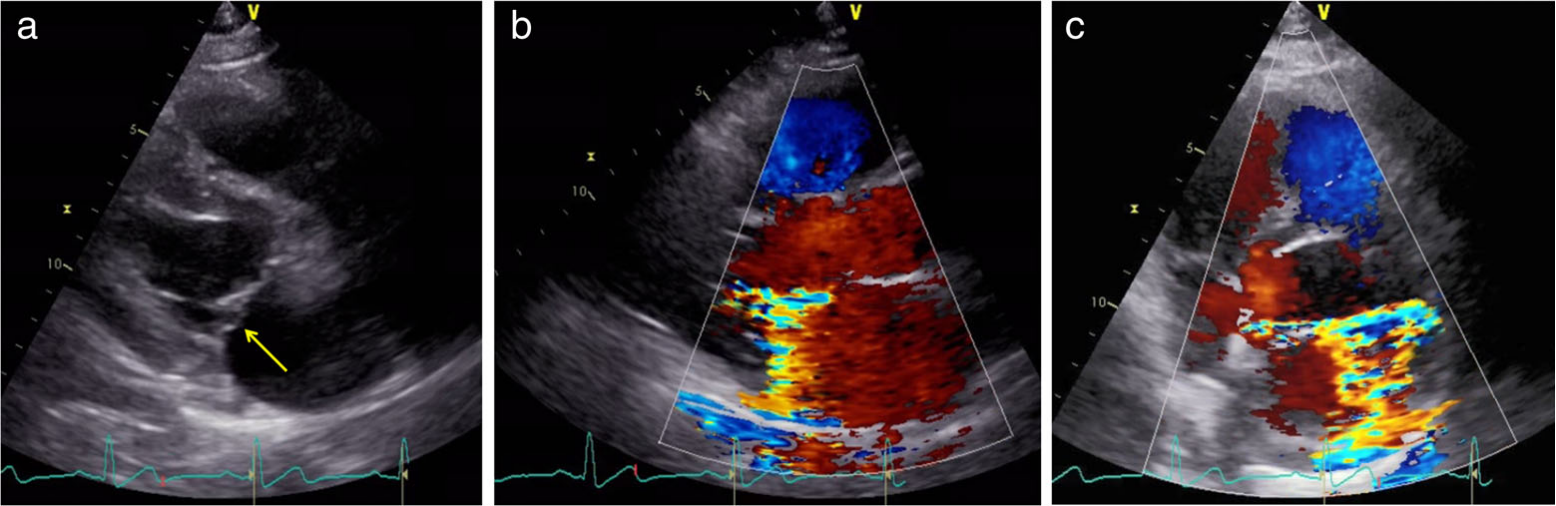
Transthoracic echocardiogram. a, b and c. Parasternal long a and b and short c axis view of the posterior mitral valve leaflet slightly protruding into the left atrium (*arrow*) with severe eccentric mitral regurgitation
